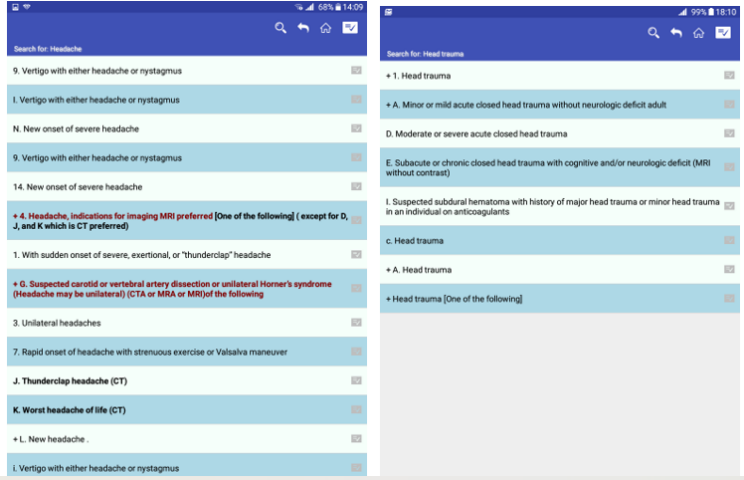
Head Computed Tomography Scan Appropriateness Criteria app search results for head trauma and headache.
